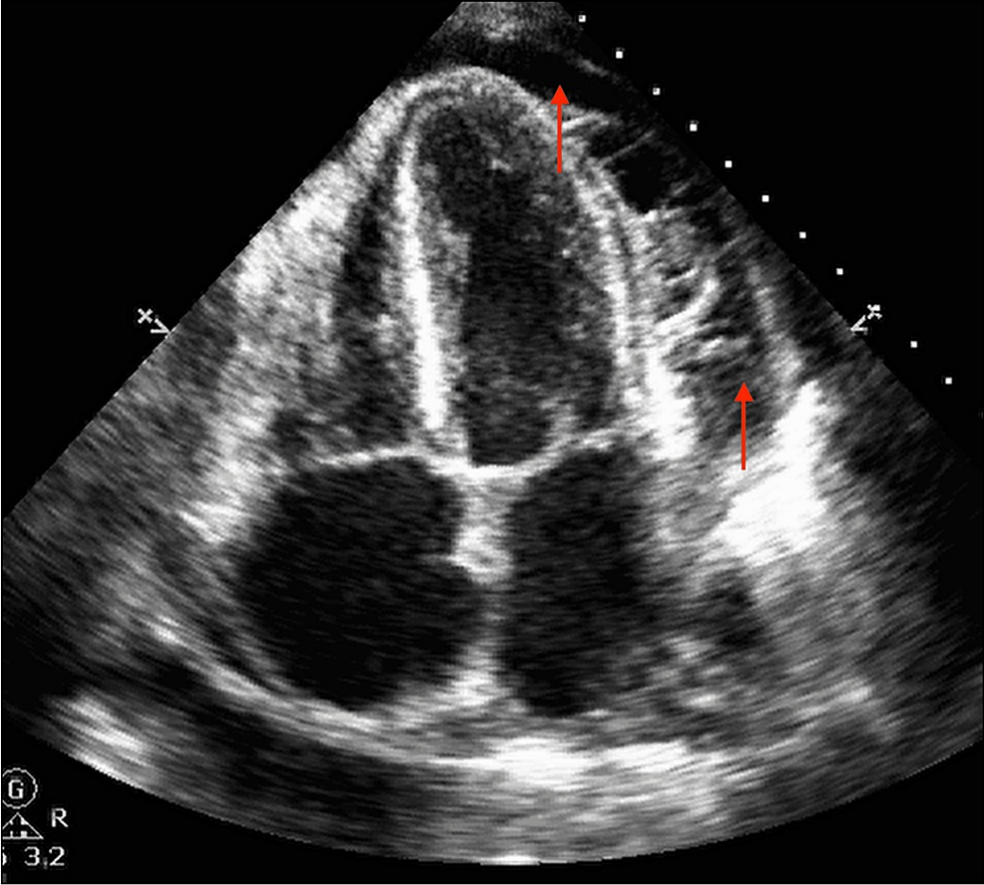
Echocardiogram in an apical four-chamber view showing a decrease in the size of the pericardial effusion after pericardiocentesis (red arrows).
